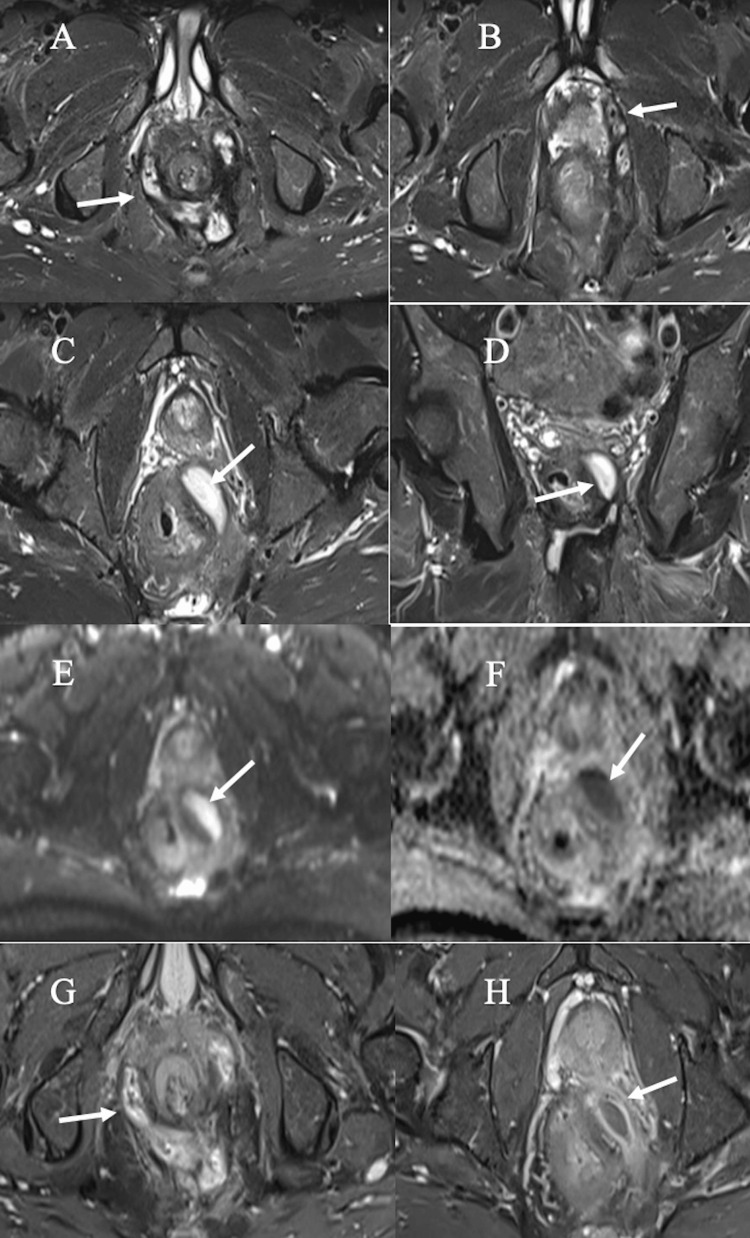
Horseshoe fistula. (A-C) Axial STIR images showing horseshoe fistula in the intersphincteric space (A) with multiple small abscesses in the intersphincteric space (B) and a well-defined abscess in left peri-rectal space (C). Coronal STIR image showing abscess in the left peri-rectal space (D). Axial DWI and ADC images showing restricted diffusion on DWI and ADC (E-F). Post-contrast fat-suppressed T1-WI showing horseshoe fistula (G) and peripheral rim enhancement in abscess (H). DWI: diffusion-weighted imaging, STIR: short tau inversion recovery; ADC: apparent diffusion coefficient
